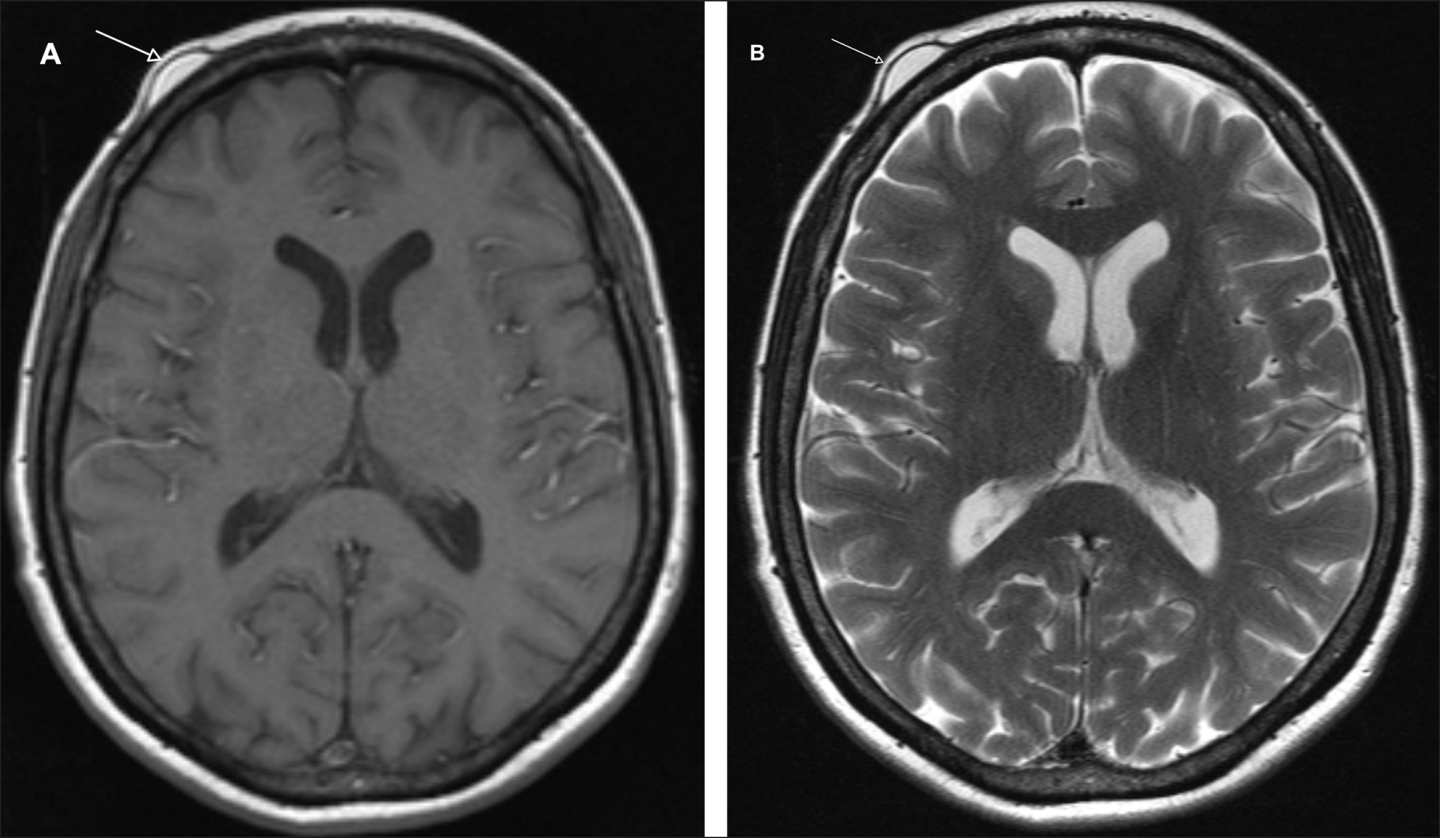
Subgaleal lipoma of the forehead. Axial T1- (A) and T2-weighted (B) sequences of head MRI showing a lipoma as a hyperintense mass located under the galea aponeurosis (arrow).
